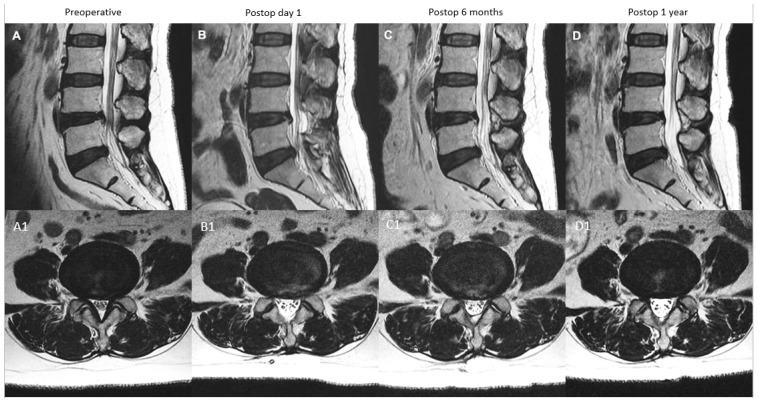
MRI appearance of remodeling patterns at preoperative, postoperative day 1, postoperative 6 months and postoperative 1 year with mid-sagittal cut in the top row and corresponding axial cut in the bottom row. Type A: continuous type, preoperative spinal stenosis at L4/5 (A,A1) with increased spinal canal area on postoperative day one MRI (B,B1). There was modest restenosis that occurred at postoperative 6 months (C,C1), which was maintained at postoperative one year with overall improved spinal canal area (D,D1).
